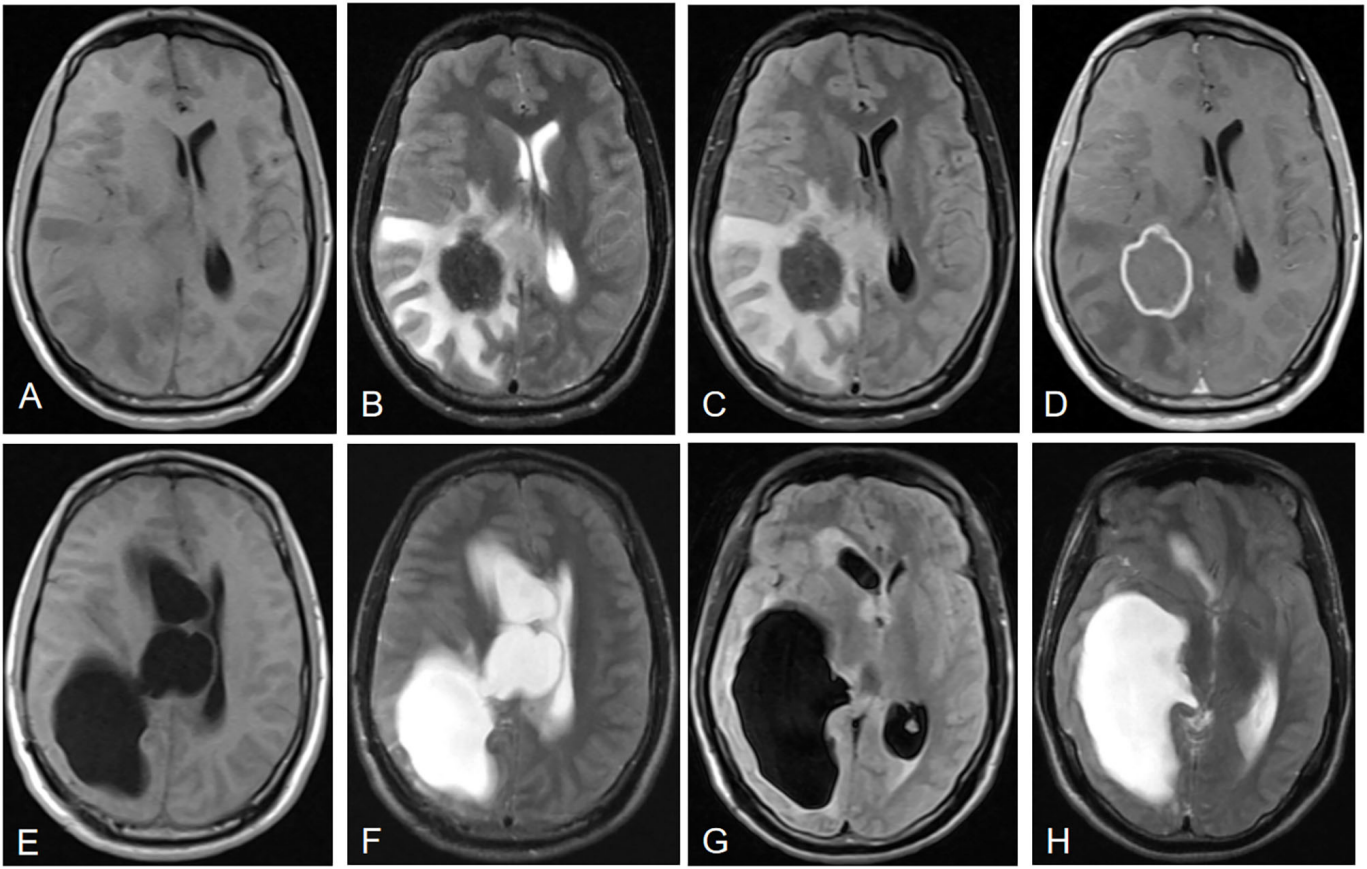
Neuroimaging features of IAE. Case 3: (A) T1-weighted imaging shows a slightly higher signal mass. (B) T2-weighted imaging reveals a heterogeneous low signal mass with peripheral high signal intensity, suggesting edema. (C) Fluid-attenuated inversion recovery imaging (FLAIR) reveals a heterogeneous low signal mass. (D) Postcontrast axial T1-weighted imaging shows cauliflower peripheral gadolinium-contrast enhancement at the lesions. (E–H) Right lateral ventricular dilatation and hydrocephalus occurred 7 months after right parietal and occipital lobe resection.
